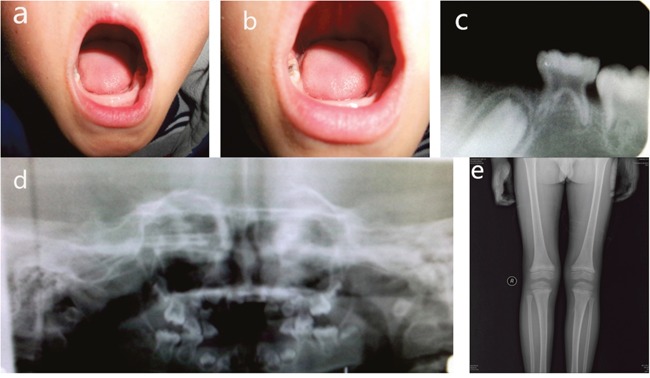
Clinical features of younger brother (A, B) Intra-oral photograph of proband showing premature exfoliation of primary teeth. (C, D) Panoramic radiographs showing reduced alveolar bone, enlarged pulp chambers, and abnormal morphology of the unerupted permanent molars. (E) Plain films of a knee joint presenting with metaphyseal osteoporosis changes.
